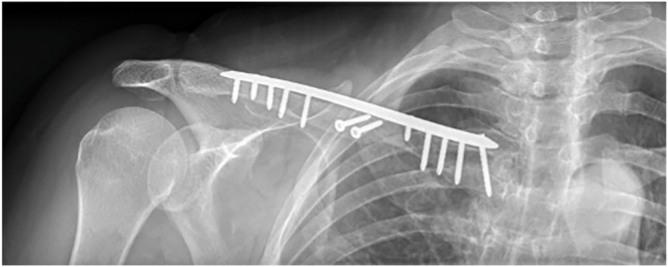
Frontal view post-operative radiograph showing correction of the hypertrophic callus and superior plate-and-screw osteosynthesis.
